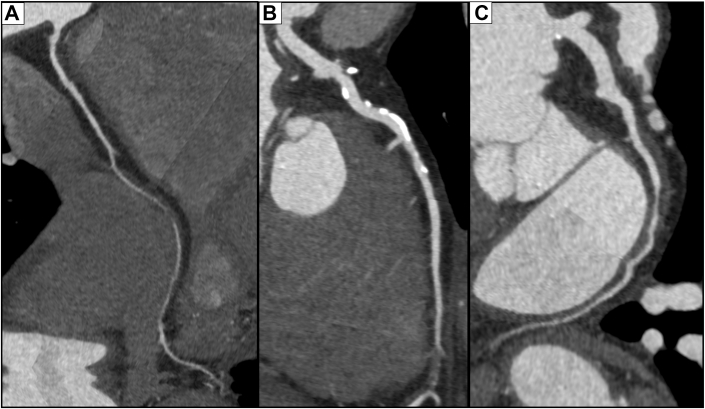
Findings on Coronary Computed Tomography Coronary CT showed noncalcified stenosis in the (A) RCA, (B) LAD, and (C) LCx, with intimal calcifications in the proximal LAD. CT = computed tomography; LAD = left anterior descending artery; LCx = left circumflex artery; RCA = right coronary artery.
